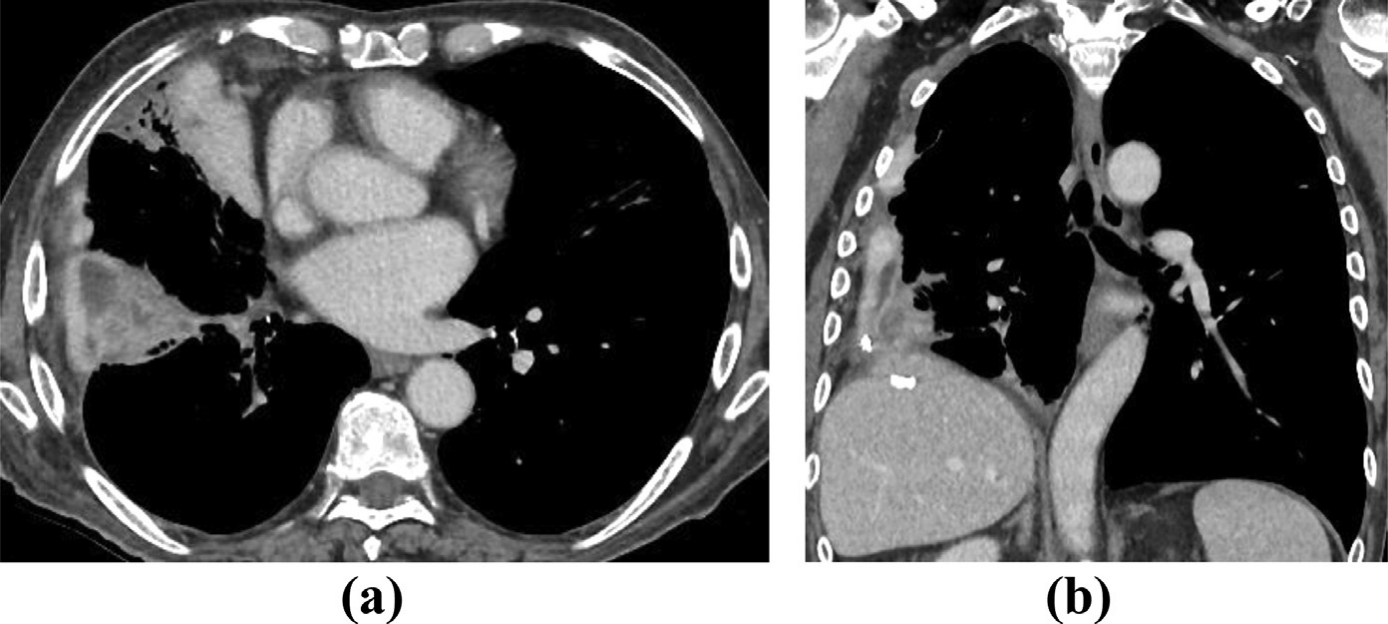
CT scan thorax (axial and coronal) shows significant improvement of pleural and parenchymal nodular masses with residual pleural thickening containing a focus of calcification.
